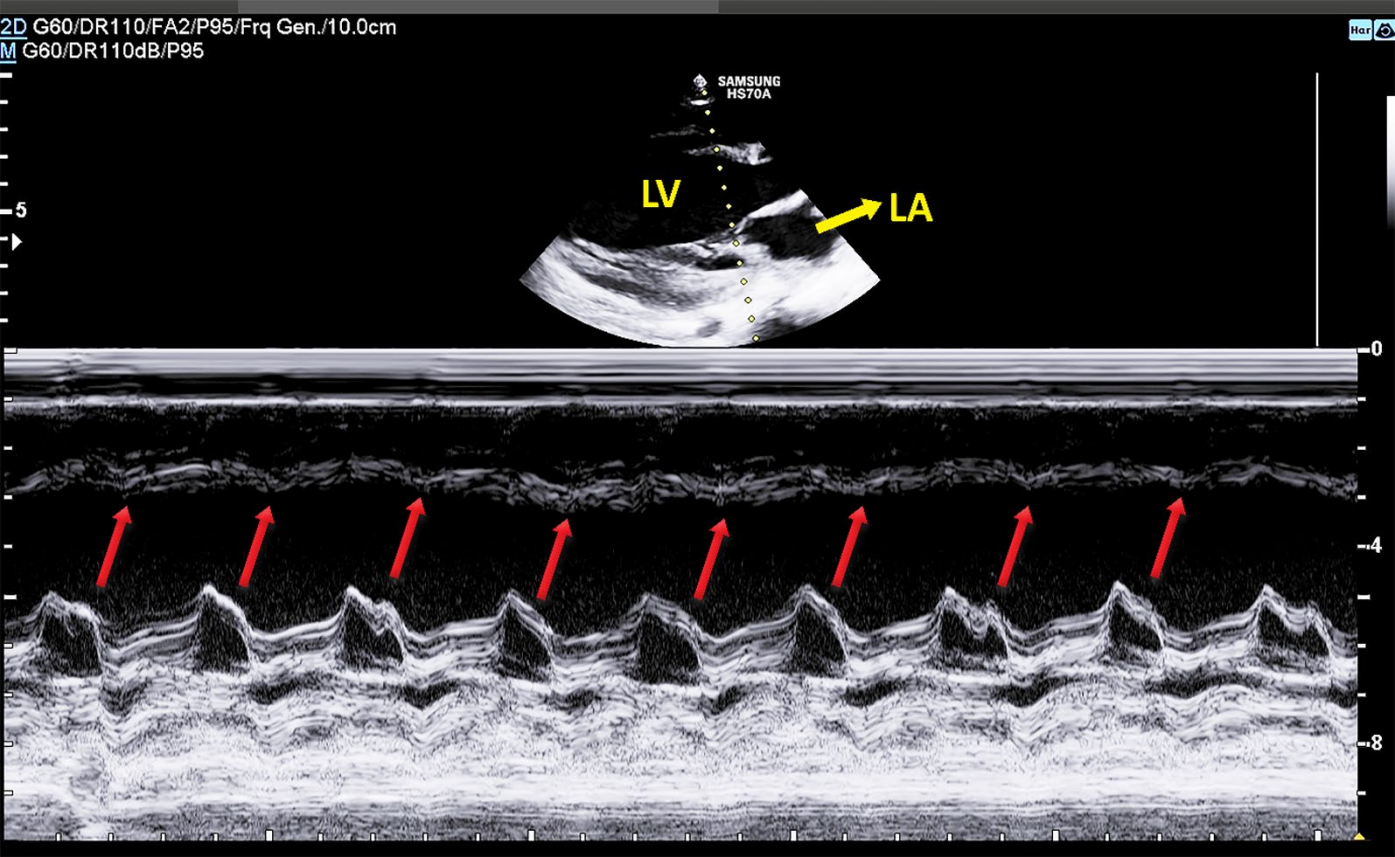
Septal flash on M-mode echocardiography of the patient
